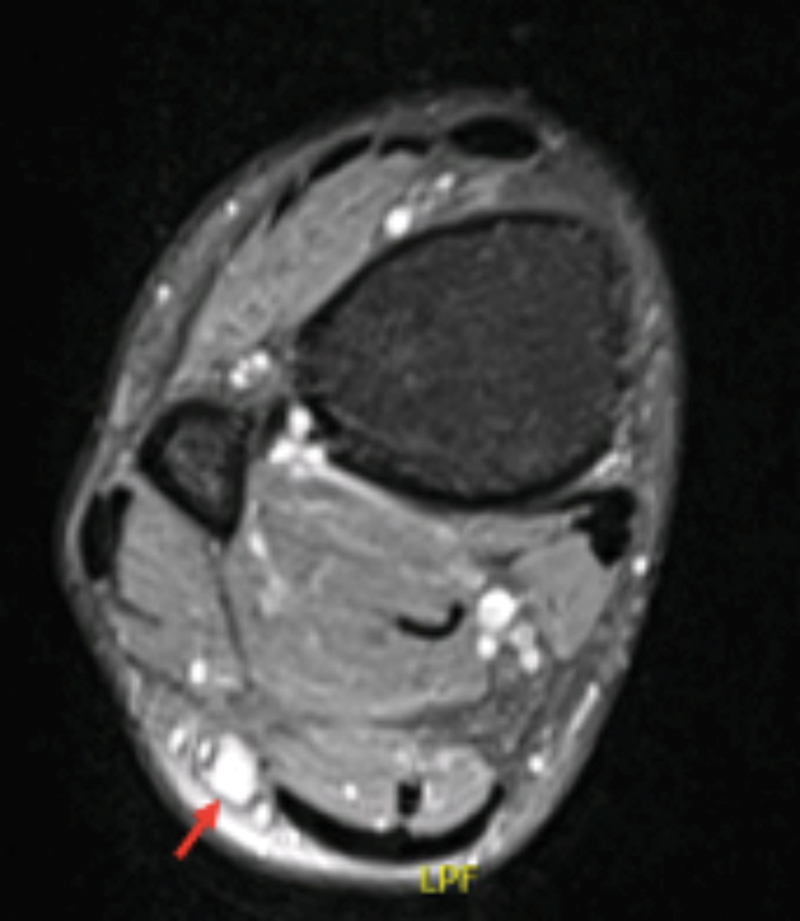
Magnetic resonance imaging (MRI) scan revealed hypeintensity on T2.
